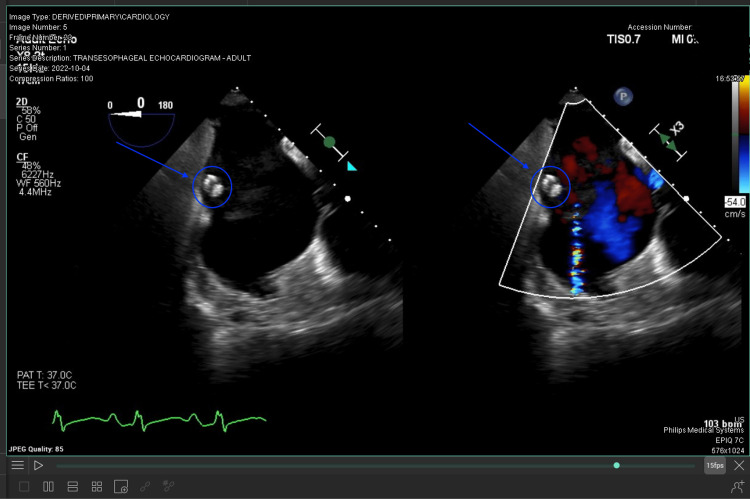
View of a calcified right atrial thrombus via transesophageal echography.
